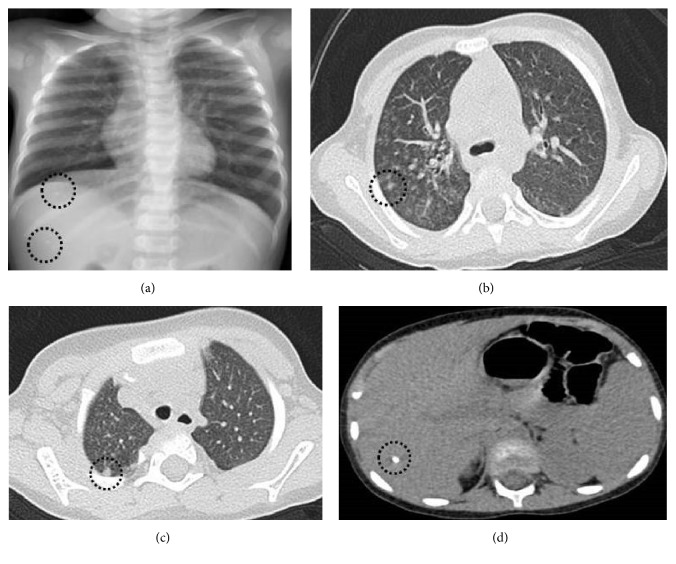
(a) Chest X-ray: bilateral infiltrations, microcalcifications in chest and abdomen. (b) and (c) Thorax CT: multiple, parenchymal nodules in both lungs, the biggest one 5 mm in diameter. (d) Thorax CT, the slices passing liver: a calcific nodule (At 26 months old).
